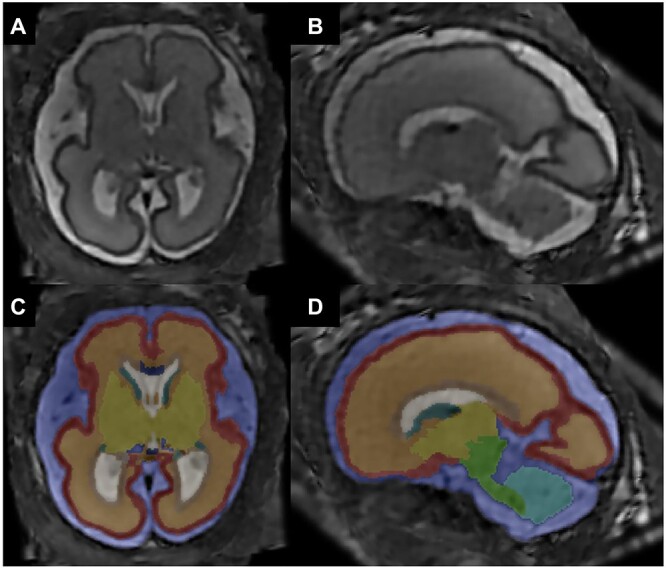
MRI super-resolution reconstruction and atlas-based tissue segmentation. A, B) Postprocessed T2-weighted MRI super-resolution reconstructions in axial (left) and sagittal (right) planes of a fetus at 26+6 gestational weeks (GW). C, D) Respective manually corrected atlas-based tissue segmentation. Color coding: blue—external CSF-spaces, red—cortex, orange—subcortical parenchyma (including the subcortical layers between, but not including, the cortical plate and subventricular), brown—periventricular zone (including the ventricular and the subventricular zone), dark green—ganglionic eminence, white—ventricular system, dark blue—corpus callosum, yellow—deep gray nuclei (basal ganglia and thalamus), light green—brainstem, light blue—cerebellum, turquoise—left hippocampus, and gray—right hippocampus.
